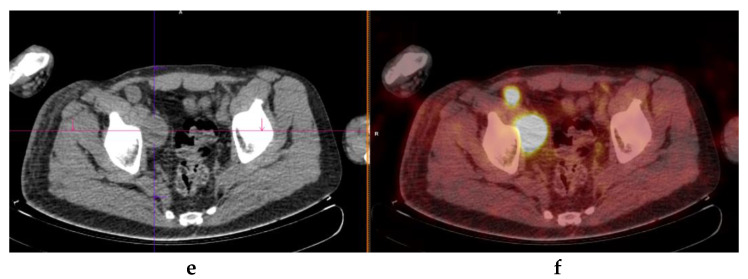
Classic KS. An FDG PET/CT showed a large hypermetabolic cutaneous lesion extending to adjacent muscles (SUV_max_ 18.6) confirmed by MRI, pathological analysis, and associated with lymph node involvement. (a) CT transaxial slice; (b) PET transaxial slice; (c) Fused PET/CT transaxial slice; (d) MIP PET image; (e) CT transaxial slice: right iliac enlarged lymph nodes; (f) Intensely hypermetabolic lymph nodes in fused PET/CT transaxial slice.
